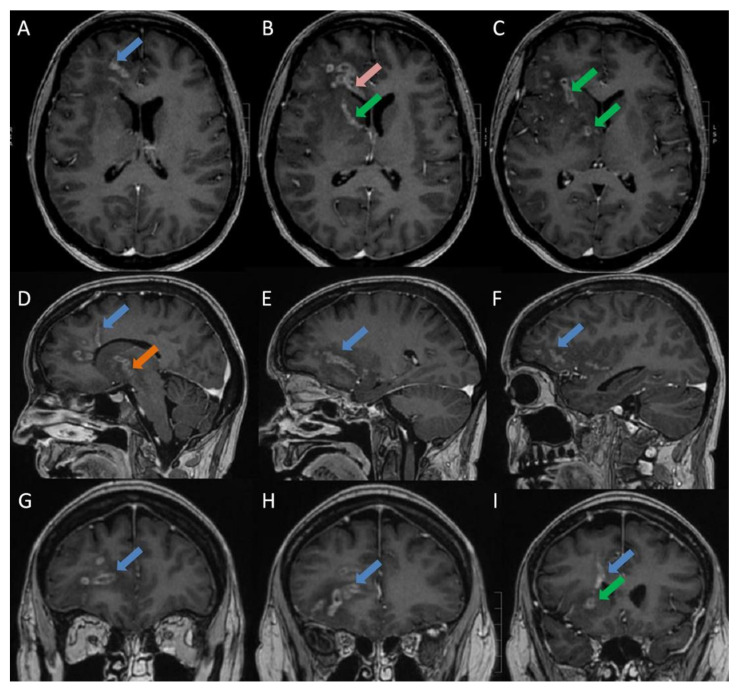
Initial MRI brain at admission: T1 BRAVO post contrast sequences—Axial (A–C: cranial to caudal), Sagittal (D–F: medial to lateral) and Coronal (G–I: anterior to posterior): shows multiple elongated peripherally enhancing lesions in the right frontal lobe (blue arrows), the right half of the genu of corpus callosum (pink arrow), the anterior limb of the right internal capsule (green arrows) as well as the right external capsule and insula, tracking along the expected location of white matter tracts and U- fibers also involving the right cerebral peduncle and right side of mesencephalon (orange arrow). No lesions were observed in the left cerebral hemisphere or in the cerebellum. No lepto- or pachymeningeal enhancement. No enhancement occurred along the cranial nerves.
